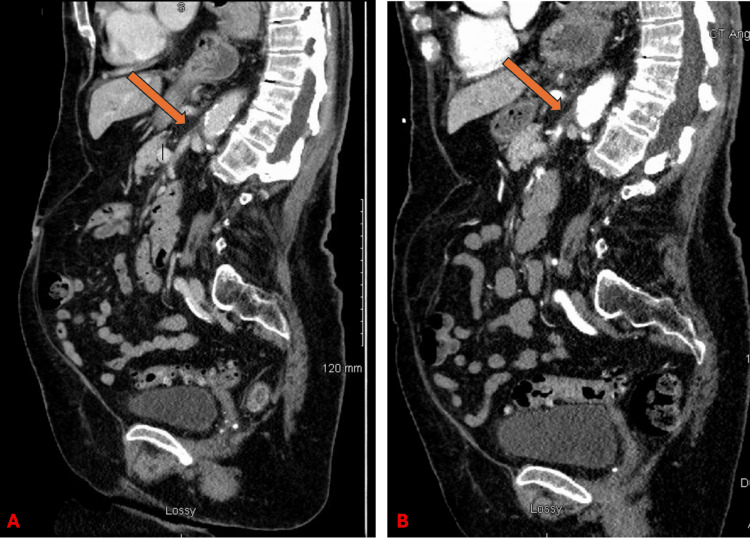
Sagittal section of a CT of the abdomen indicating retrograde filling (A) and the superior mesenteric artery (B).
